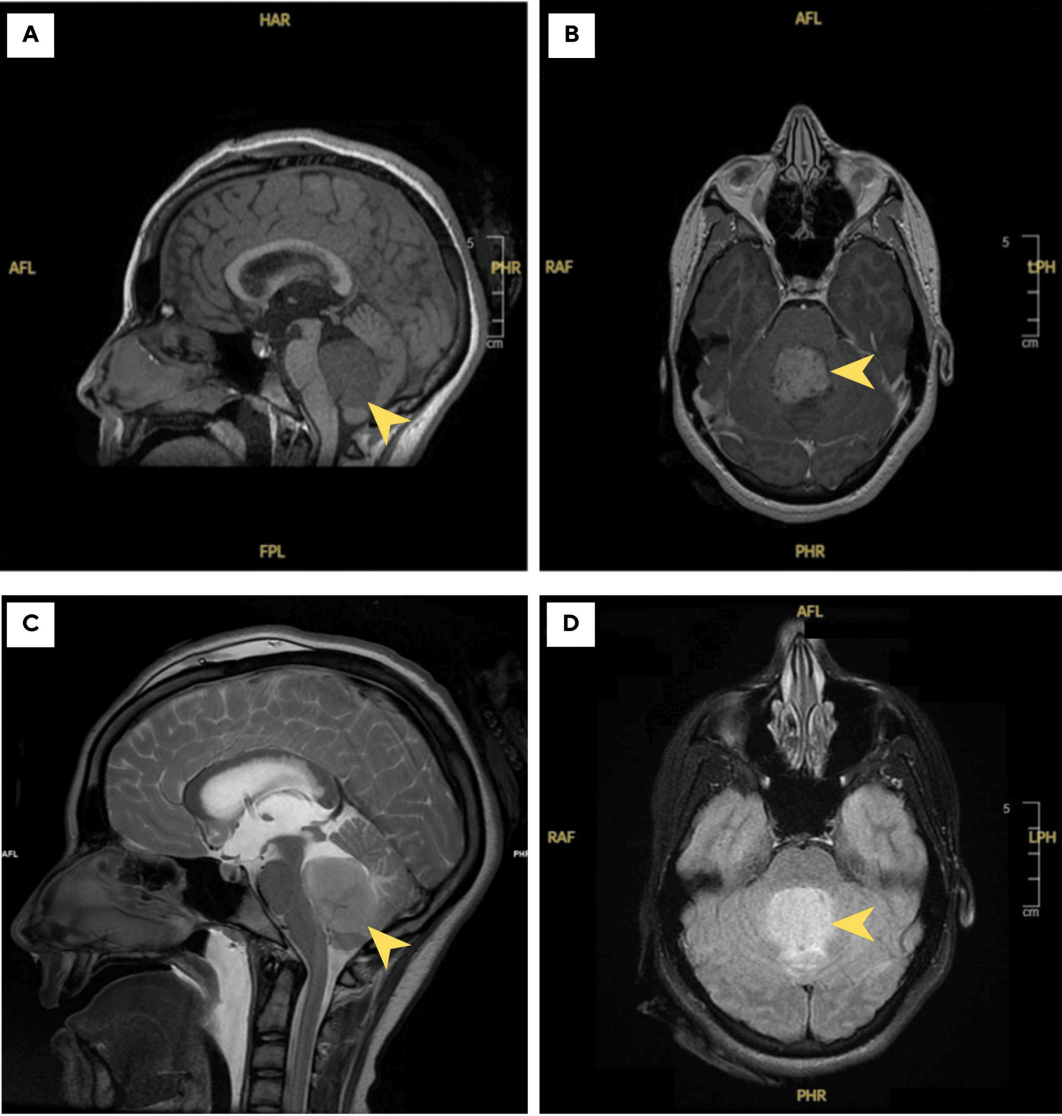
MRI images of the brain MR imaging showing a 42mm mass (yellow arrowheads) arising from the 4th ventricle, appearing hypointense to grey matter on T1w images (Sagittal scan A), with homogenous contrast enhancement (Coronal scan B) and hyperintense on T2w sequence images (Sagittal scan C) and with better delineation and increased hyperintensity on FLAIR sequence (Coronal Scan D).
